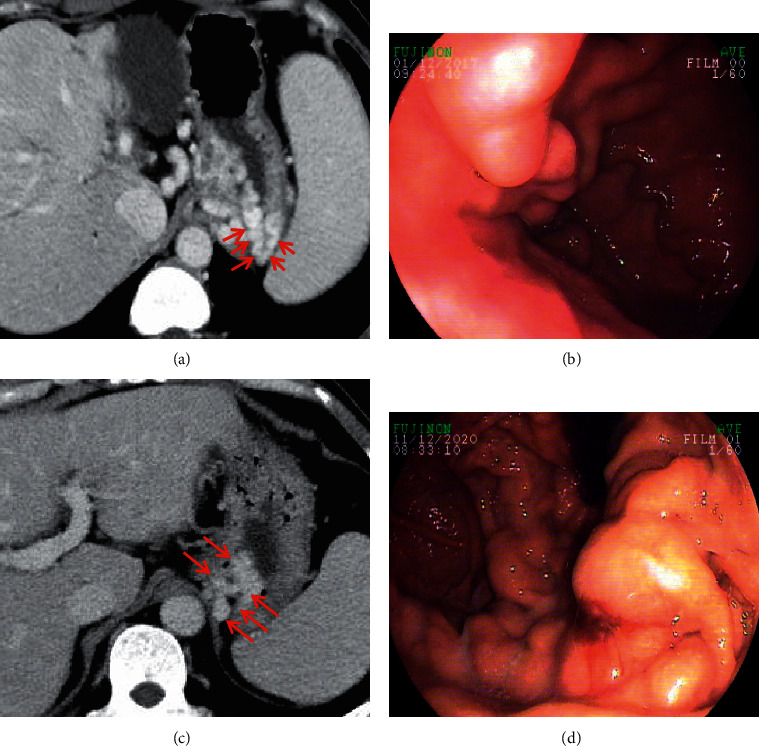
Gastric varices. In a 47-year-old female and a 56-year-old male with liver cirrhosis, the axial contrast-enhanced CT scan on the portal vein phase demonstrated that gastric varices (red arrow) were long, nodular, and tortuous enhanced channels at the gastric fundus. Upper gastrointestinal endoscopy showed dilated and tortuous varices at the gastric fundus. Note: (a, b) the contrast-enhanced CT scan and endoscopy of a 47-year-old female, respectively; (c, d) the contrast-enhanced CT scan and endoscopy of a 56-year-old male, respectively.
